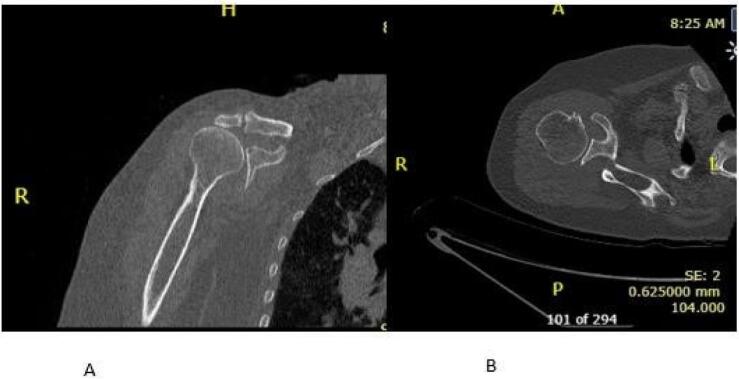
A and B (CT) scan right shoulder: superior subluxation of humeral head, moderate osteoarthritic changes involving glenohumeral joint.
